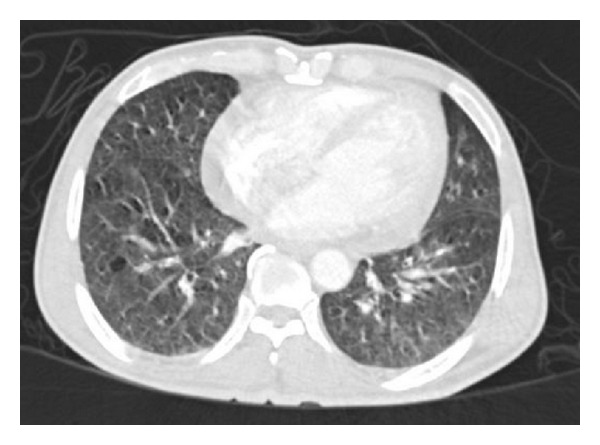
Computed tomography of chest: multiple nodular opacities in the upper lobe of right lung with interlobular interstitial thickening. Minimal pericardial effusion.
